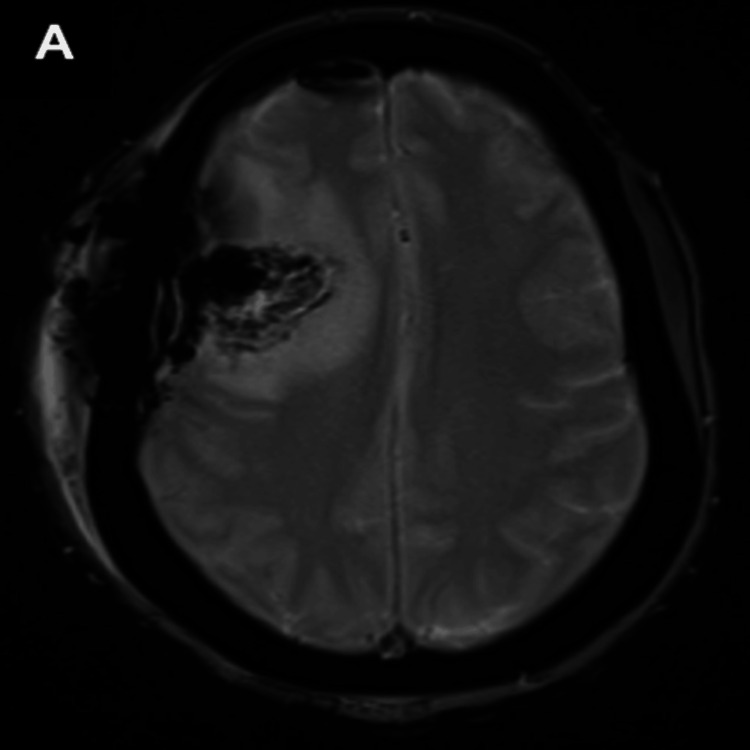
MRI showing interval post-surgical changes with fluid within the resection cavity and a thin rind of cytotoxic edema around the cavity. No overt residual mass identified and leftward midline shift slightly improved.
